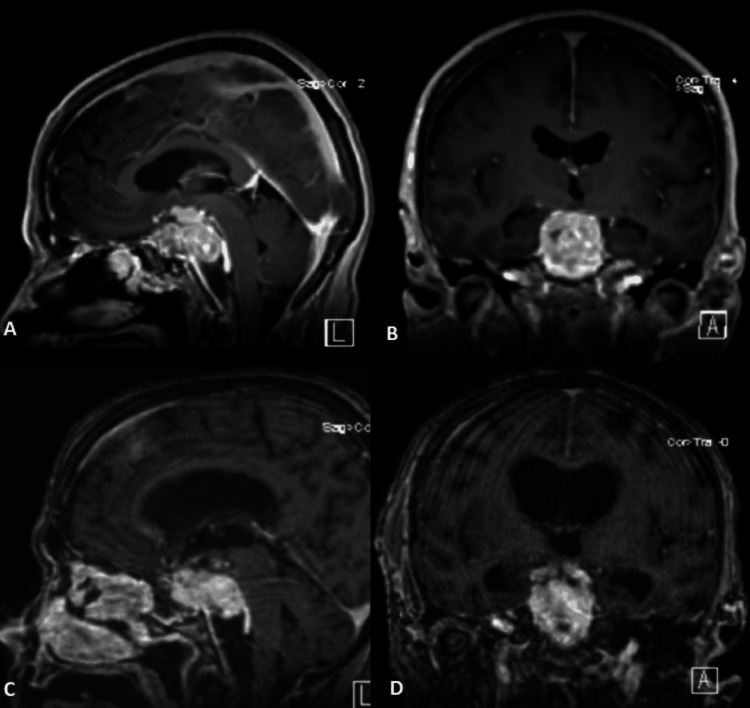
Top row: Post-endonasal approach sagittal (A) and coronal (B) T1 post-gadolinium MRI images revealed a subsequent increase of the sellar/suprasellar lesion (2.9 x 2.1 x 3.3 cm) with prepontine cisternal effacement, exhibiting aggressive growth with cavernous extension. Bottom row: Sagittal (C) and coronal (D) T1 post-gadolinium MRI images at the two-week follow-up after open craniotomy revealed continued enlargement of the residual lesion (2.6 x 3.3 x 4.0 cm) with optic nerve and brainstem compression as well as obstruction of the cerebral aqueduct with interval enlargement of the ventricular system.
